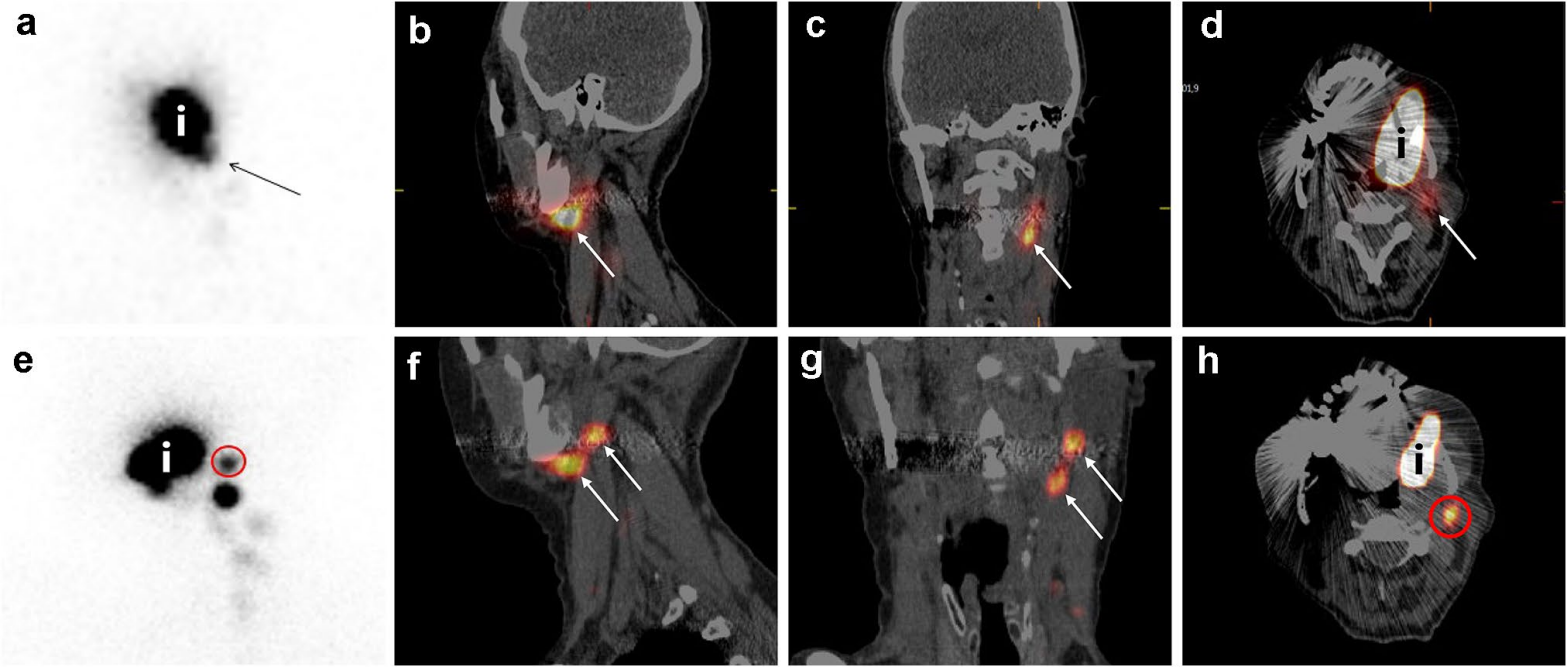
A 77 year old patient (patient 3) with a cT2N0 primary tumor in the inferior alveolar process, located on the left side. Using the streamlined protocol, two SLNs were identified (level Ib left; level IIa left). Evaluation of early static LSG (anterior-oblique view) allowed discrimination between two hotspots in level IIa. Written informed consent for publishing these images was obtained from this patient. a Planar early dynamic LSG 10 min anterior view; one hotspot level Ib left (arrow). b SPECT-CT sagittal plane; one large hotspot level IIa (arrow). c SPECT-CT coronal plane; one large hotspot level IIa left (arrow). d SPECT-CT axial plane; injection site (i) and one large hotspot level IIa left (arrow). e Planar early static LSG anterior-oblique view; one additionally identified hotspot level IIa left (red circle). f Post-evaluation SPECT-CT reconstruction* sagittal plane; discrimination between two hotspots level IIa (arrows). g Post-evaluation SPECT-CT reconstruction* coronal plane; discrimination between two hotspots level IIa left (arrows). h Post-evaluation SPECT-CT reconstruction* axial plane; more cranially localized one additionally identified hotspot (red circle). *SPECT-CT reconstructions were made with ITK-SNAP (www.itksnap.org) [17]. *LSG* lymphoscintigraphy
